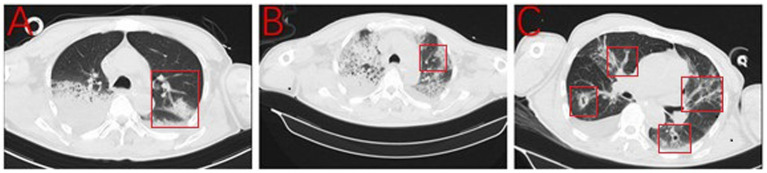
Serial chest CT findings during hospitalization. (A) Day 1: bilateral posterior high-density opacities and bilateral pleural effusion. A halo sign was observed. (B) Day 9: increased bilateral patchy high-density opacities with greater lesion extent and increased bilateral pleural effusion compared with the previous scan. Consolidation was observed. (C) Day 16: decreased lesion extent in both upper lobes and the right middle lobe, with new cavitary lesions in both lower lobes and decreased bilateral pleural effusion compared with the previous scan. An air crescent sign was observed.
